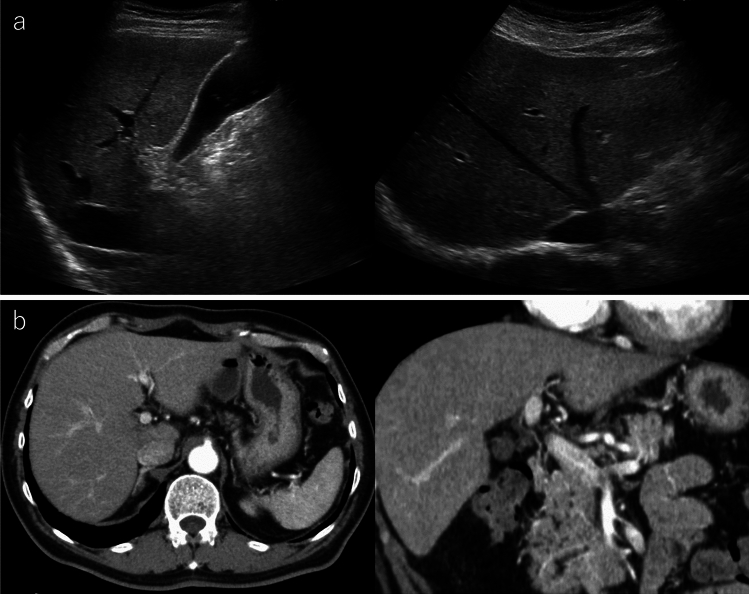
Representative images of abdominal ultrasonography and computed tomography (CT) in the present case. Images of ultrasonography (a) and CT (b) showing that the liver was not cirrhotic, but the liver edge was slightly dull
